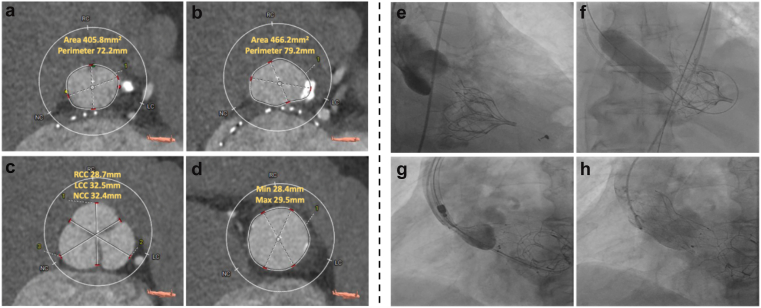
Preprocedural CT assessment and procedural fluoroscopy during TAVI. (a–d) Preprocedural multidetector computed tomography (CT) analysis: (a) aortic annulus measurements (area = 405.8 mm^2^; perimeter = 72.2 mm); (b) left ventricular outflow tract (LVOT) measurements (area = 466.2 mm^2^; perimeter = 79.2 mm); (c) sinus of Valsalva dimensions (right coronary cusp [RCC] = 28.7 mm, left coronary cusp [LCC] = 32.5 mm, noncoronary cusp [NCC] = 32.4 mm); and (d) sinotubular junction measurements (minimum diameter = 28.4 mm, maximum diameter = 29.5 mm). Multiplanar reconstructions were additionally reviewed to demonstrate preserved clearance between the Tendyne frame and aortic annulus, supporting a low risk of LVOT obstruction. (e–h) Fluoroscopic images during transcatheter aortic valve replacement (TAVR): (e) baseline aortogram; (f) balloon aortic valvuloplasty with a 22 mm True balloon; (g) partial deployment of a 27 mm Navitor valve; and (h) final fully deployed Navitor 27 mm valve in optimal position. Abbreviation: TAVI, transcatheter aortic valve implantation.
